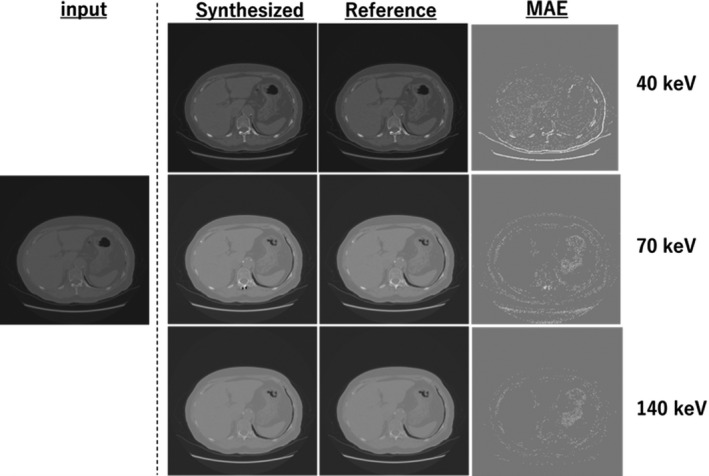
Samples obtained from monoenergetic CT image generation at the abdominal level: input image is the equivalent kV‐CT image, synthetic and reference images are the monoenergetic CT images at 40, 70, and 140 keV, and MAE is the difference between the synthesized and reference monoenergetic CT images.
